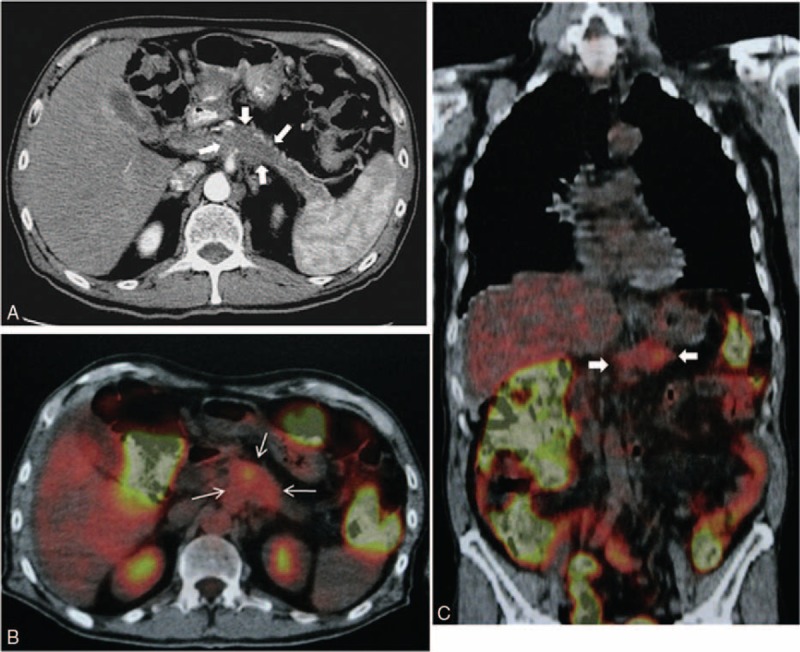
A, CT scan showing an ill-defined tumor in the pancreatic body (arrows). B and C, PET scan (axial and coronal images) showing abnormal uptake of F18-FDG in the site of the pancreatic tumor (arrows).
